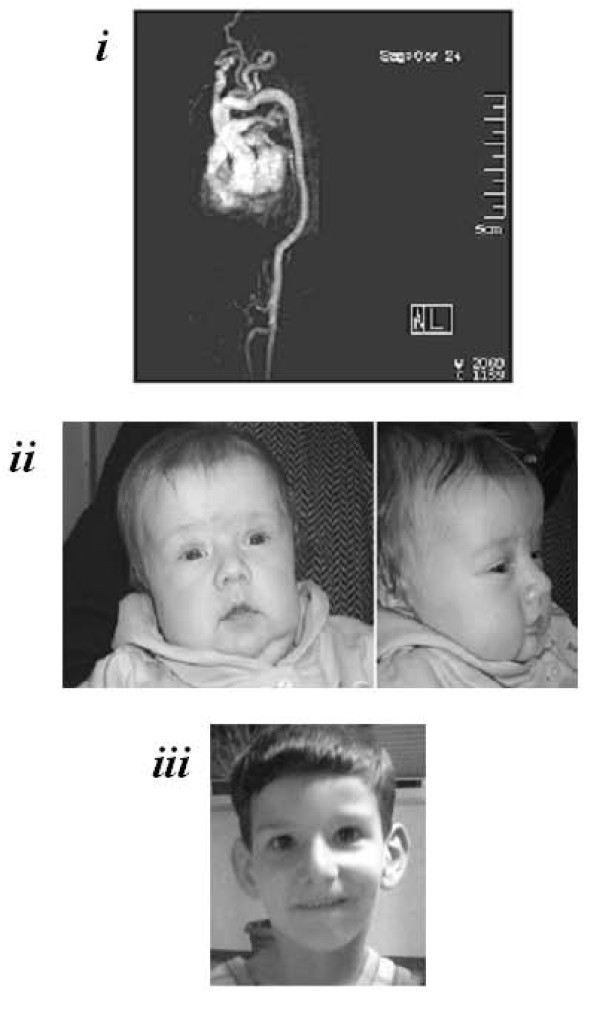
Radiographic and facial features of the two Patients. *i) *MRA of Patient 1 showed tortuosity and kinking of aortic arch, mild hypoplasia of descending and abdominal aorta, tortuosity of all the sovraortic trunks with kinking of left common carotid and left subclavian arteries, ectasia of the innominate artery origin, and bilateral reduction of peripheral pulmonary branches. *ii) *Patient 1 showed elongated face, micrognathia, mild blepharophimosis, downslanting palpebral fissures, beaked nose, high-arched palate. *iii) *Patient 2 showed elongated face, low-set and anteverted ears, down-slanting palpebral fissures, high nasal bridge.
